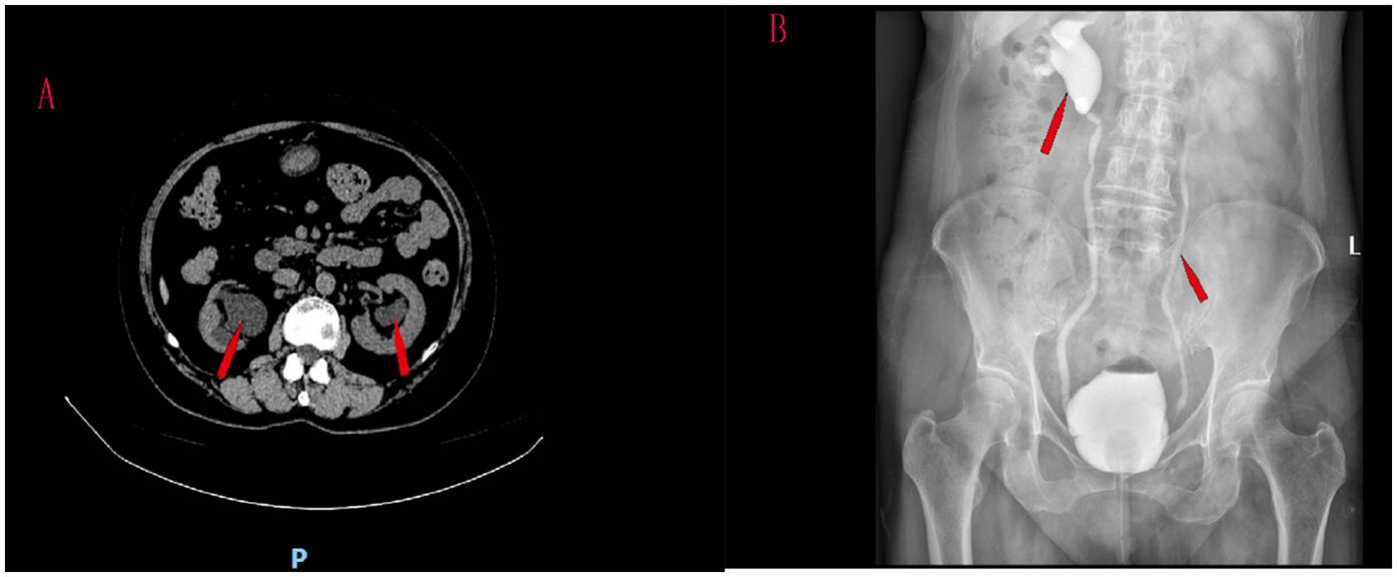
CT scan showed bilateral ureteral hydrodilation (A) reflux grade was V in the right ureter and III in the left ureter (B).
